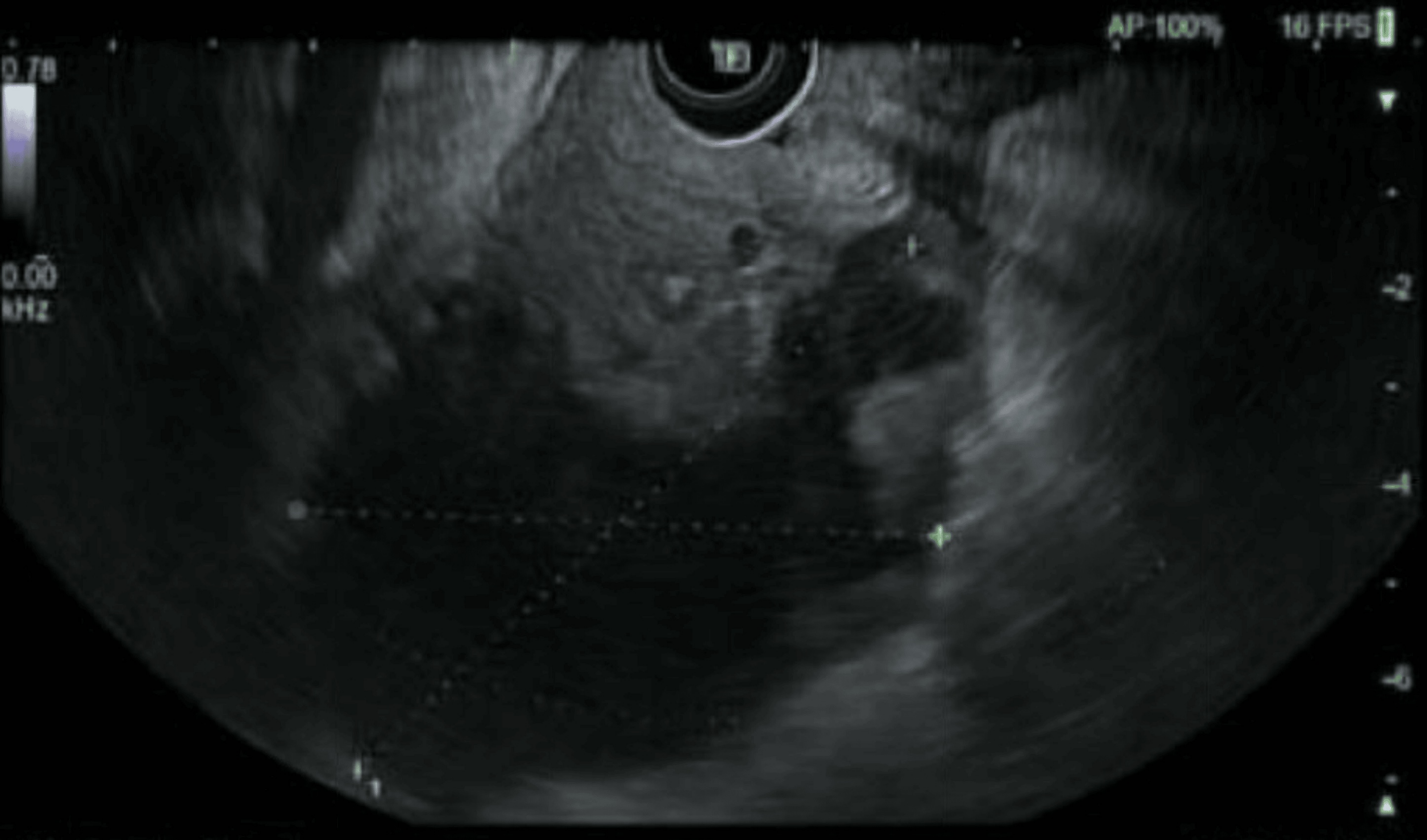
Endoscopic ultrasound of duodenal mass with hypoechogenicity and septations
